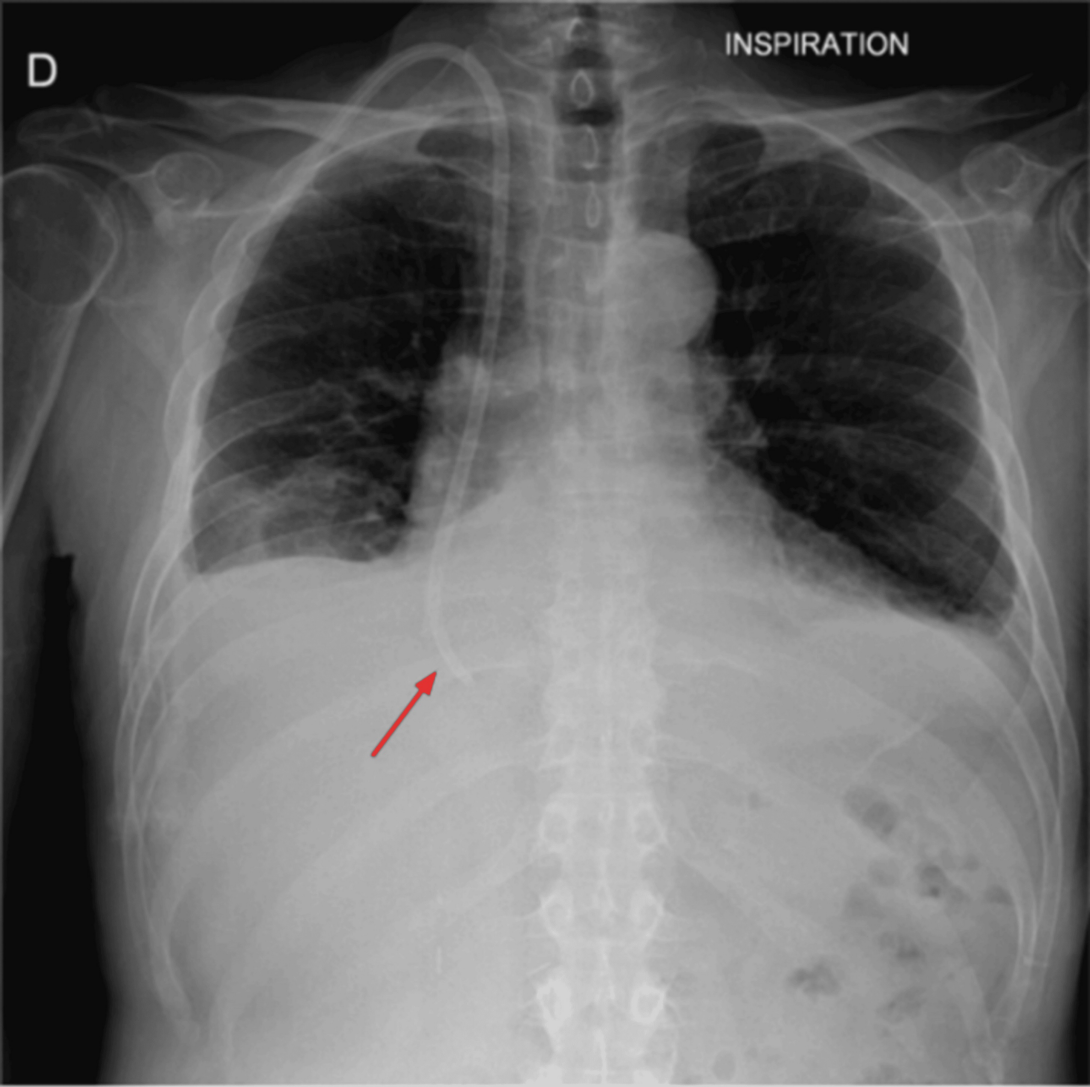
Post-procedural chest radiography. Post-procedural chest radiograph confirming intracardiac placement of the catheter (red arrow).
